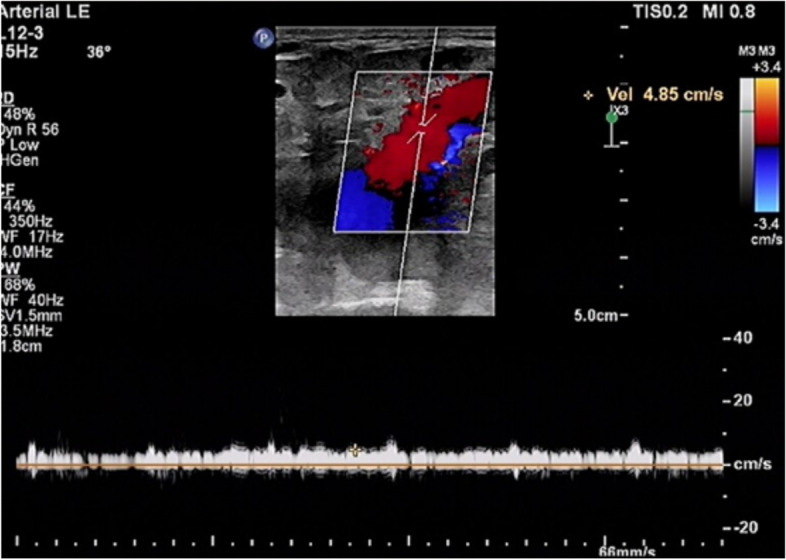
Spectral Doppler of the left great saphenous vein showed continuous
